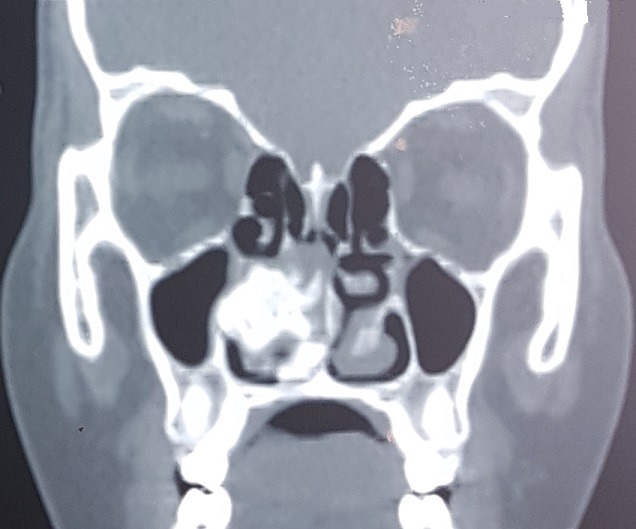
Coronal sinonasal computed tomography (CT) showing a high-density, calcified mass located between the inferior turbinate and nasal septum in the right nasal cavity with destruction of medial wall of maxillary sinus
